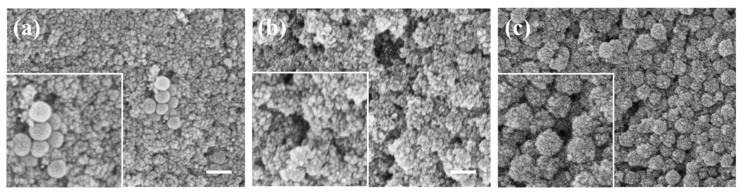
Products obtained by different dispersion methods: (a) Product obtained by mechanical stirring dispersion method; (b) product obtained by ultrasonic dispersion method; (c) product obtained by simultaneous ultrasonic and mechanical stirring dispersion method (scale bar: 200 nm).
